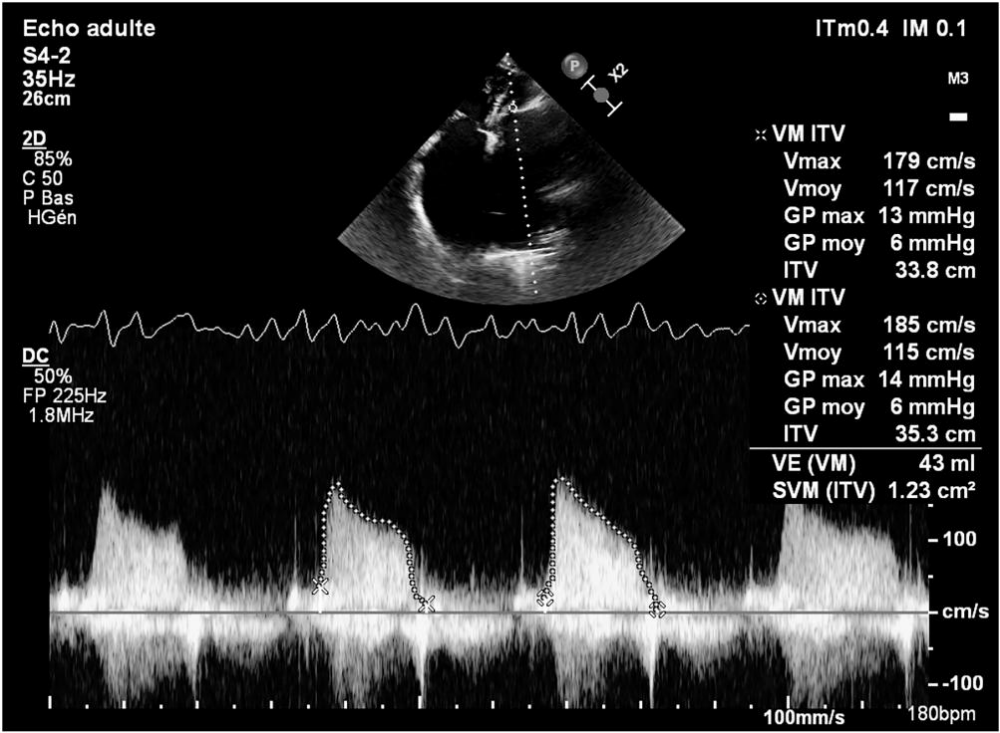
Transthoracic echocardiogram apical four-chamber view assessing the medium transmitral gradient, which measures 6 mmHg.
